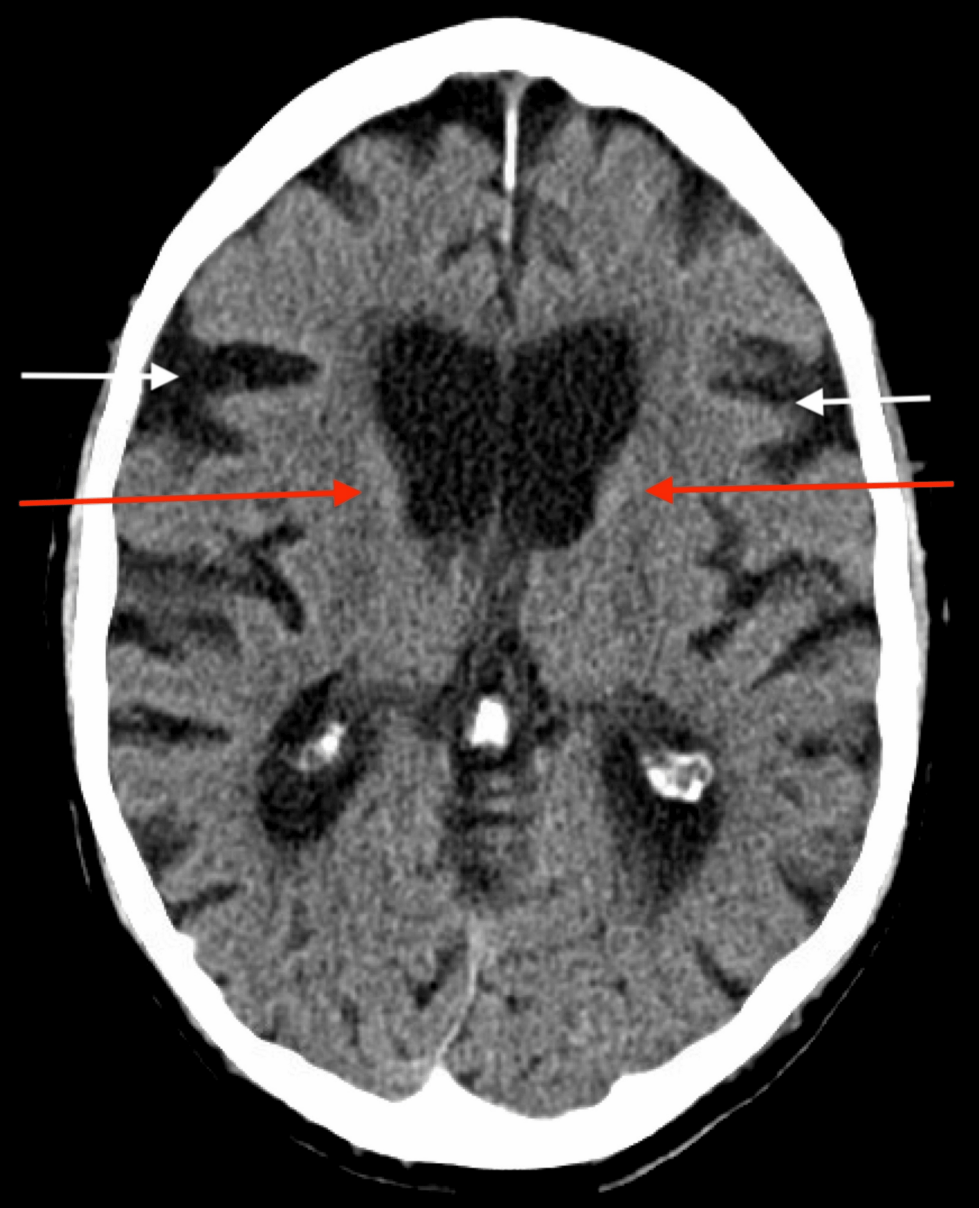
CT of brain, showing cerebral atrophy (white arrows) along with mild periventricular and subcortical microvascular ischemic white matter changes (red arrows) CT: computed tomography
